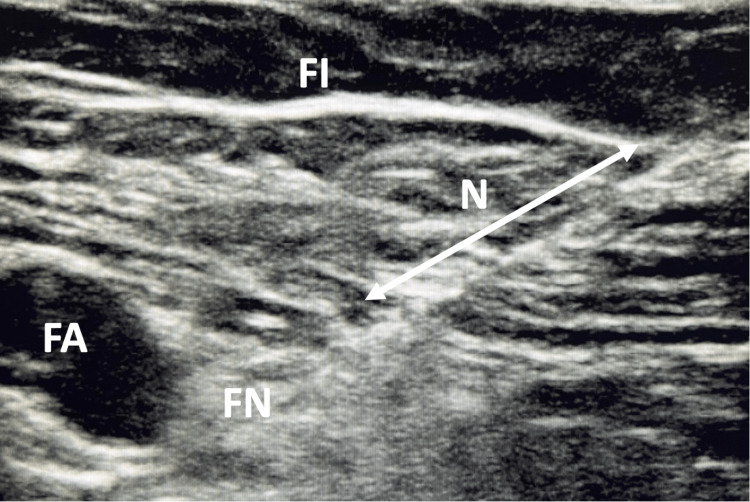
Transverse ultrasound image of the femoral nerve (FN), and artery (FA) The needle (N) was advanced medially and a single dose of 10 ml of 0.25% ropivacaine and 10 ml of lidocaine 1% with 1:200,000 epinephrine was injected between the fascia iliaca (FI) and femoral nerve (FN)
